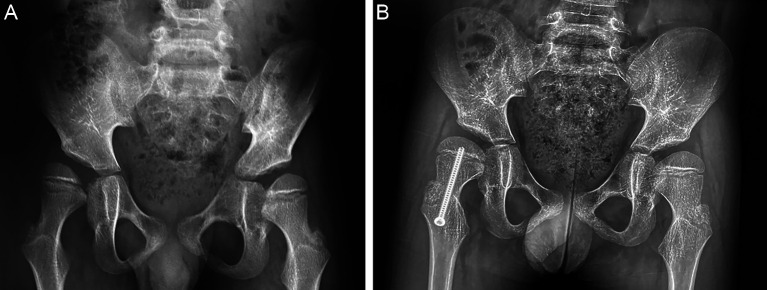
(A) Preoperative radiograph of a 4-year-old patient with spastic quadriplegia and dystonia. Hip abduction was 20° on the right side and 45° on the left side. (B) One year after STR and growth control on the right hip there was improvement of the morphology of the femoral head and acetabulum. Hip abduction was symmetric at 45°.
